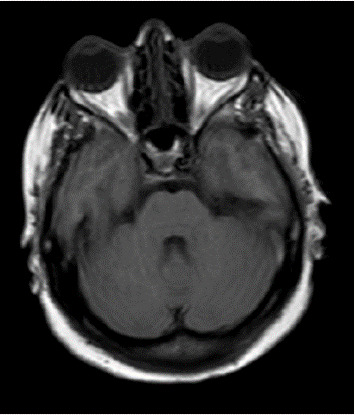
A section of the patient's T1-weighted MRI scan.
